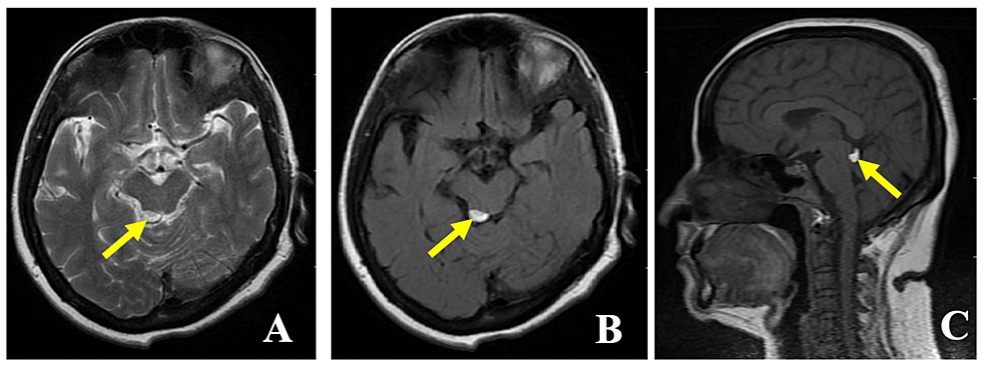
Non-contrast MRI brain images: A) T2 axial section showing a lipoma in the quadrigeminal cistern, B) Fluid-attenuated inversion recovery (FLAIR) axial section showing a lipoma in the quadrigeminal cistern, C) T1 Sagittal section showing a lipoma in the quadrigeminal cistern
